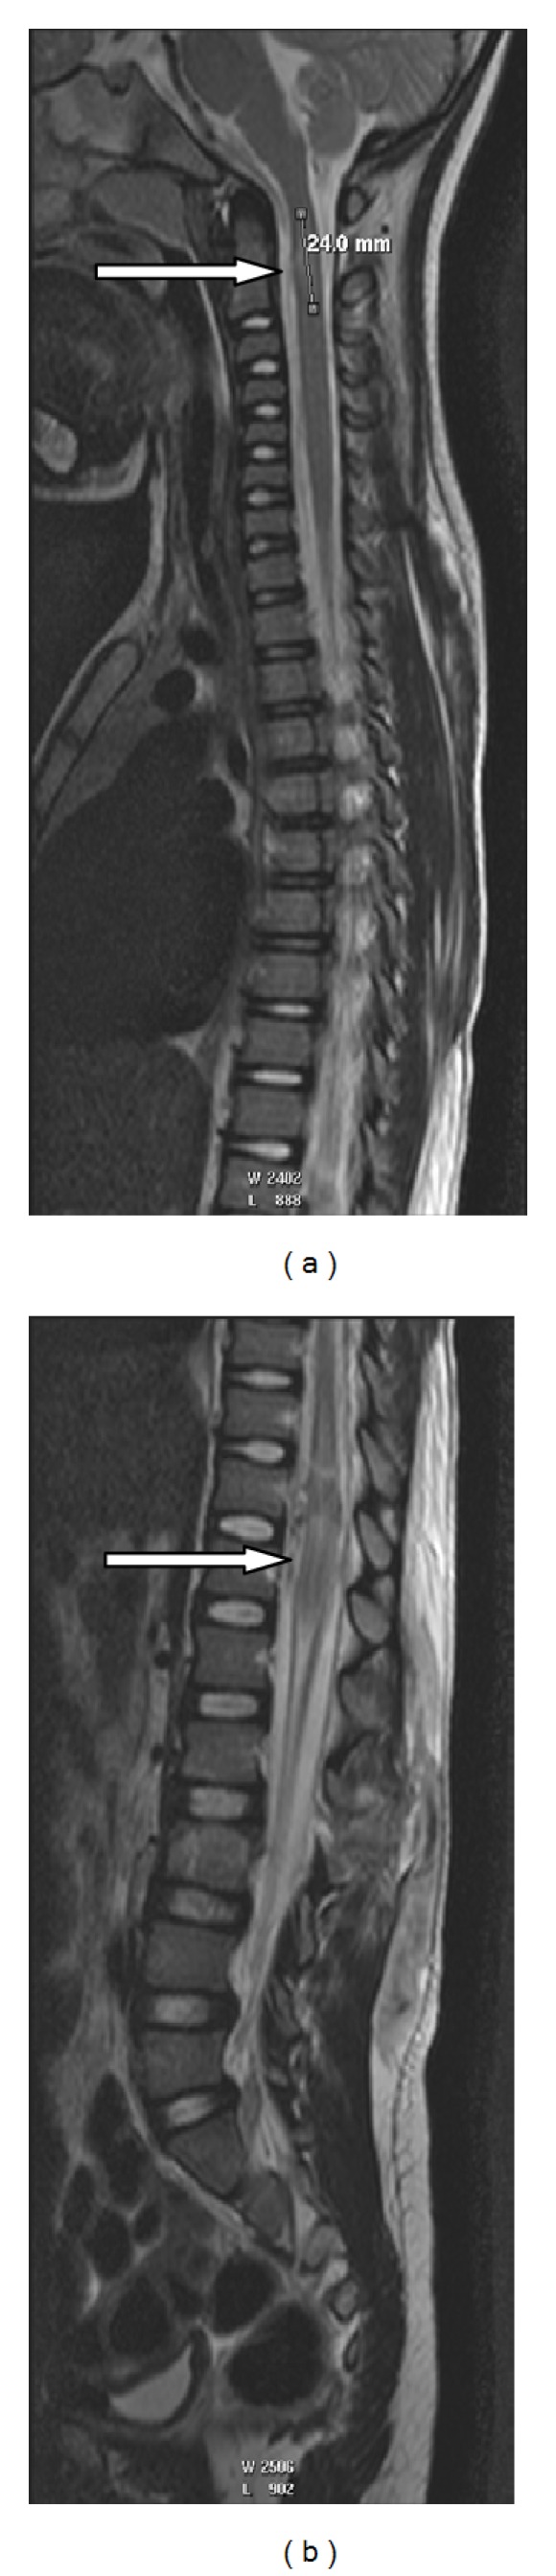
Lateral MRI images of the spinal cord at the cervical level (a) and the lumbar level (b). The spinal injuries are indicated with white arrows.
